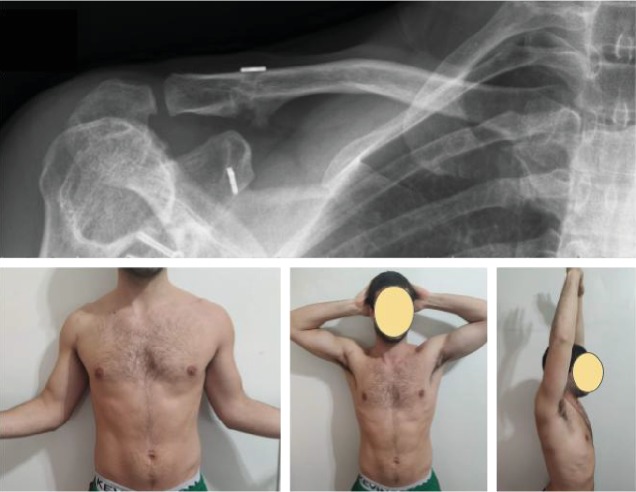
10 weeks after the procedure, radiographic union, and clinical assay. Full shoulder range of motion had been achieved, and shoulder strength was near 100%.
